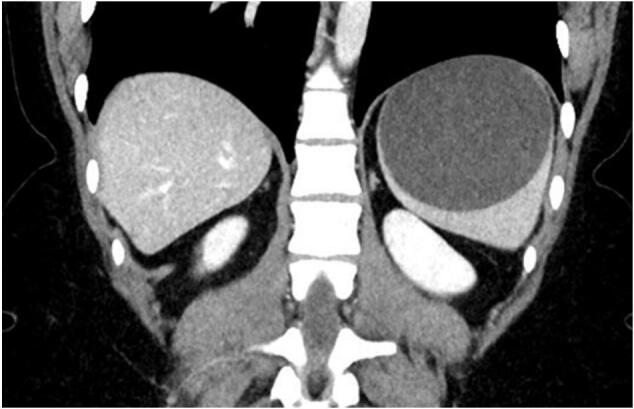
Sagittal view of the splenic cyst on CS CT scan.
